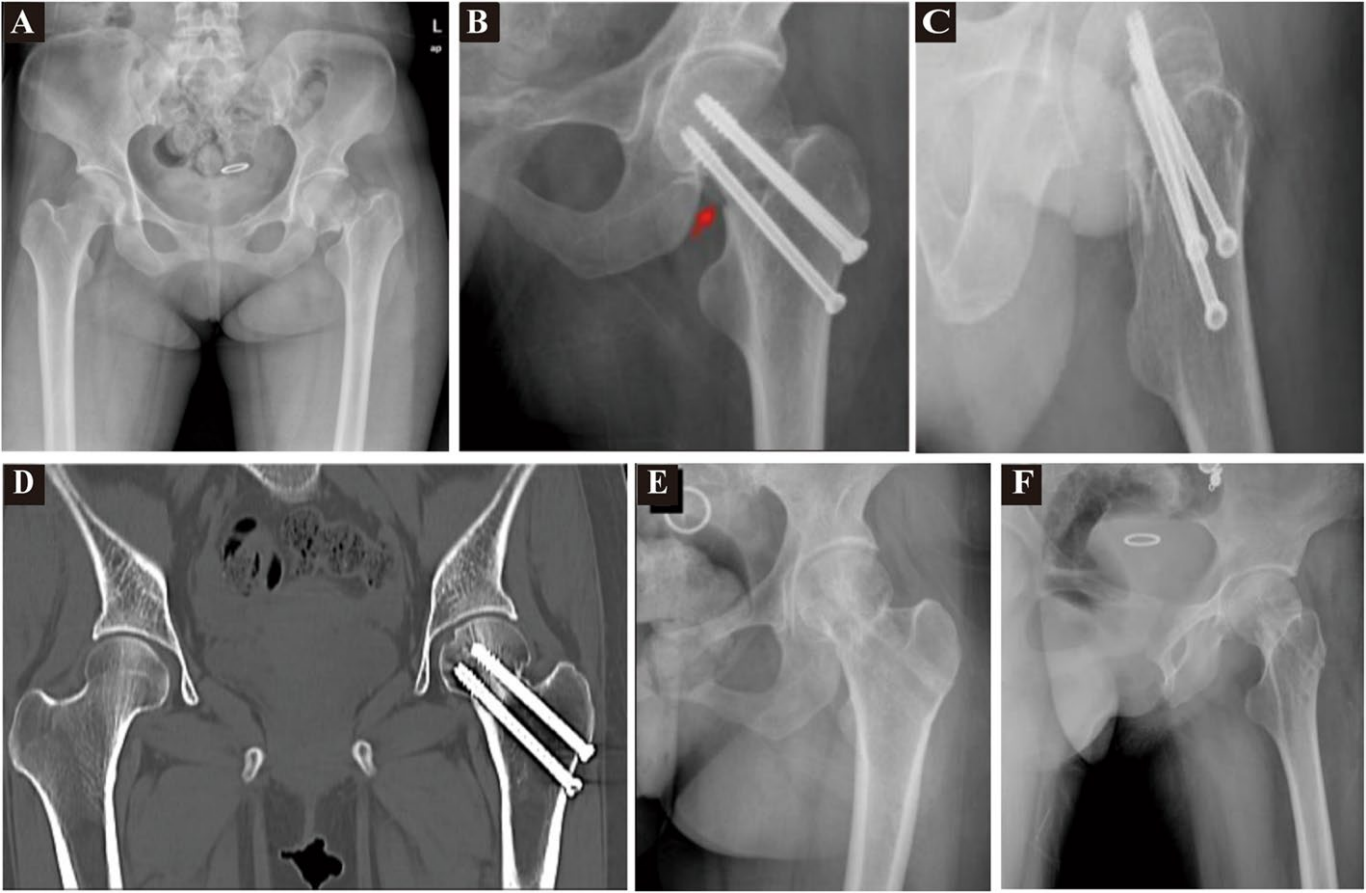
A 55-year-old female with a femoral neck fracture. A femoral neck fracture double hip positive-position X-ray; B and C postoperative and lateral X-ray of femoral neck fracture (positive support at the red arrow); D 1 year after the operation of femoral neck fracture, double hip CT showed complete bone healing of the fracture; E and F: 18 months after surgery, the femoral neck fracture was treated with internal fixation and the positive and lateral radiographs were removed. The fracture was completely fixed and healed, and no obvious necrosis and collapse of the femoral head was observed
